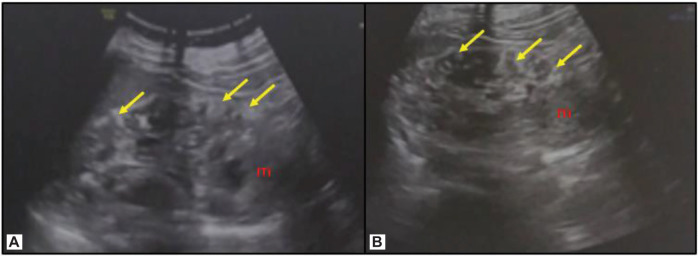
(A, B) Ultrasound images of the left kidney reveal multiple elongated echogenic focal lesions close to the cortex, suggesting angiomyolipomas. Note the mass from the lower pole (m).
